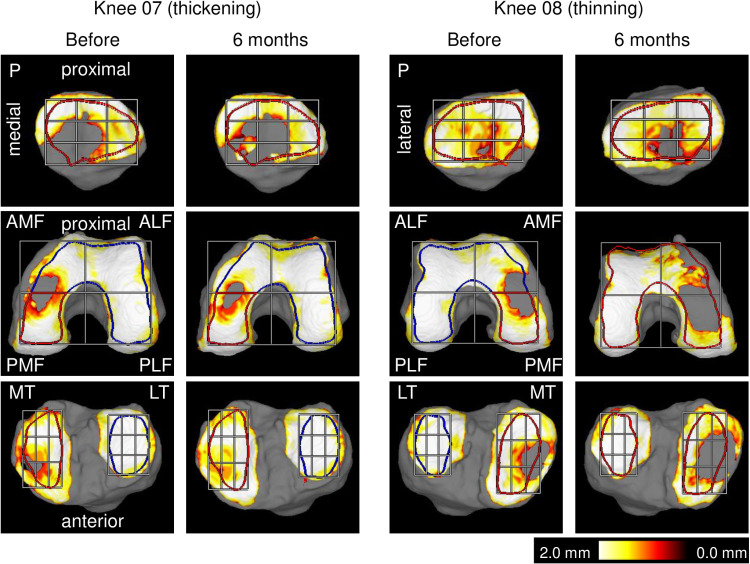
Reconstructed 3D MRI for knee cartilage and ROIs for quantification of cartilage thickness. Knee 07 is a representative case in which the cartilage thickness increased in the PMF and MT regions. Knee 08 is a representative case in which the cartilage thickness decreased in the PMF and MT regions. P, patellar; AMF, anterior medial femoral; ALF, anterior lateral femoral; PMF, posterior medial femoral; PLF, posterior lateral femoral; MT, medial tibial; LT, lateral tibial. Note: The P, MT, and LT regions are divided into 9 sections for reference. The patella is inverted mediolaterally to show its relationship with the femur.
